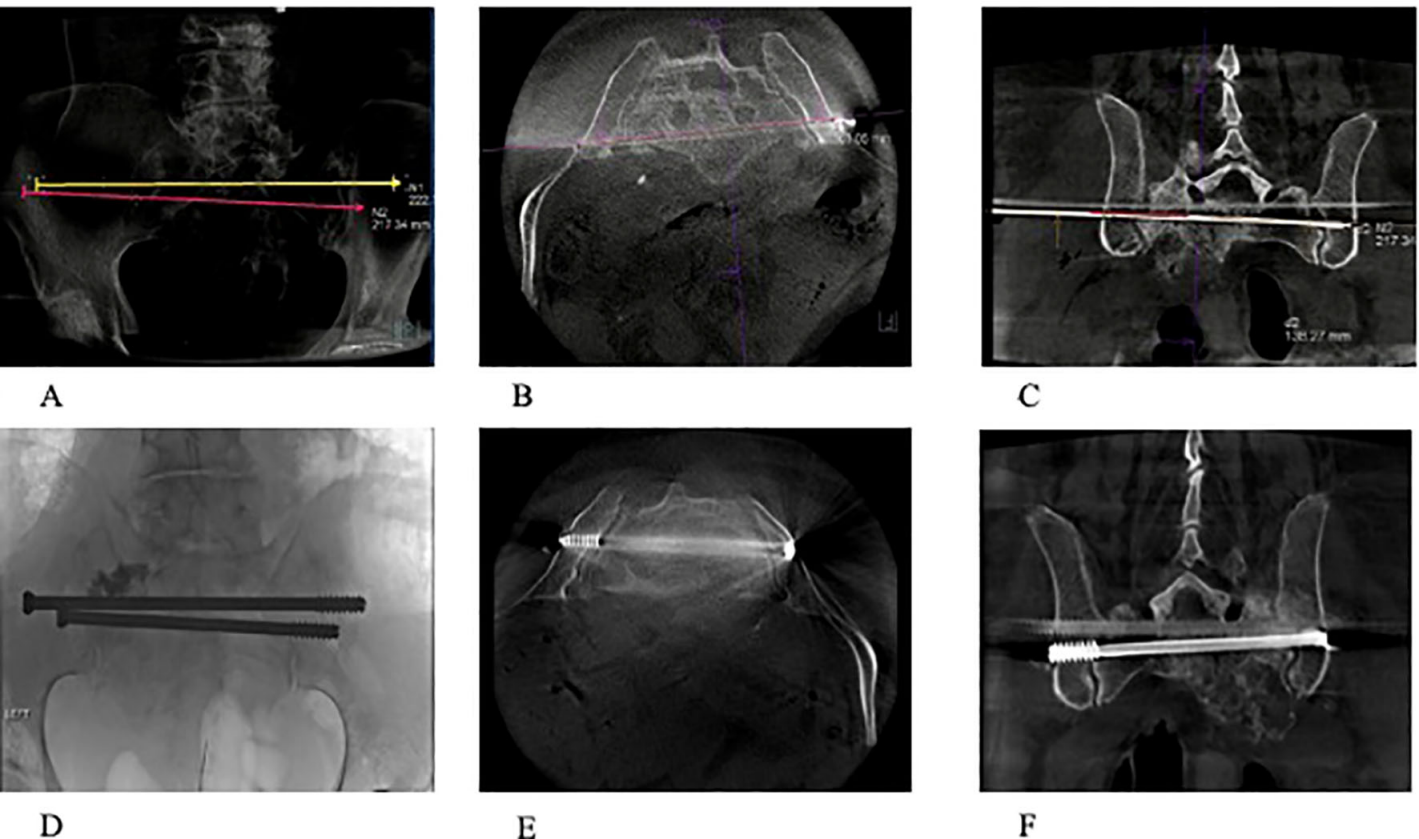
(A–F) A 74-year-old patient with osteoradionecrosis of pathological fracture secondary to metastatic pancreatic cancer. (A–C) Radiograph and CT scans (axial and coronal) showing the planned screw trajectories through S1 and S2 corridors. (D–F) Completion radiograph and CT scans (axial and coronal) demonstrating stabilization of the sacrum with the use of percutaneous trans-sacral screw fixation and sacroplasty.
